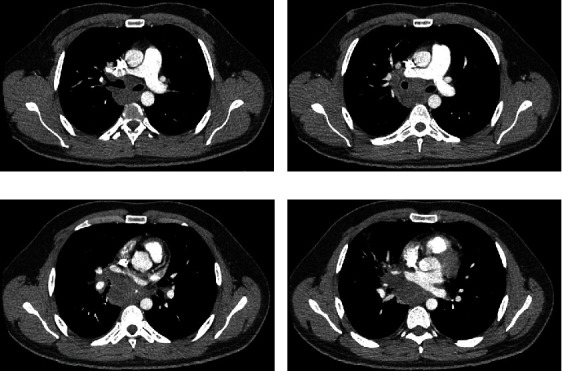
(a–d) CT scan of the chest demonstrating a large subcarinal mass encasing the right middle lobe bronchus. (c) Subtle perforation just distal to the right middle lobe orifice.
